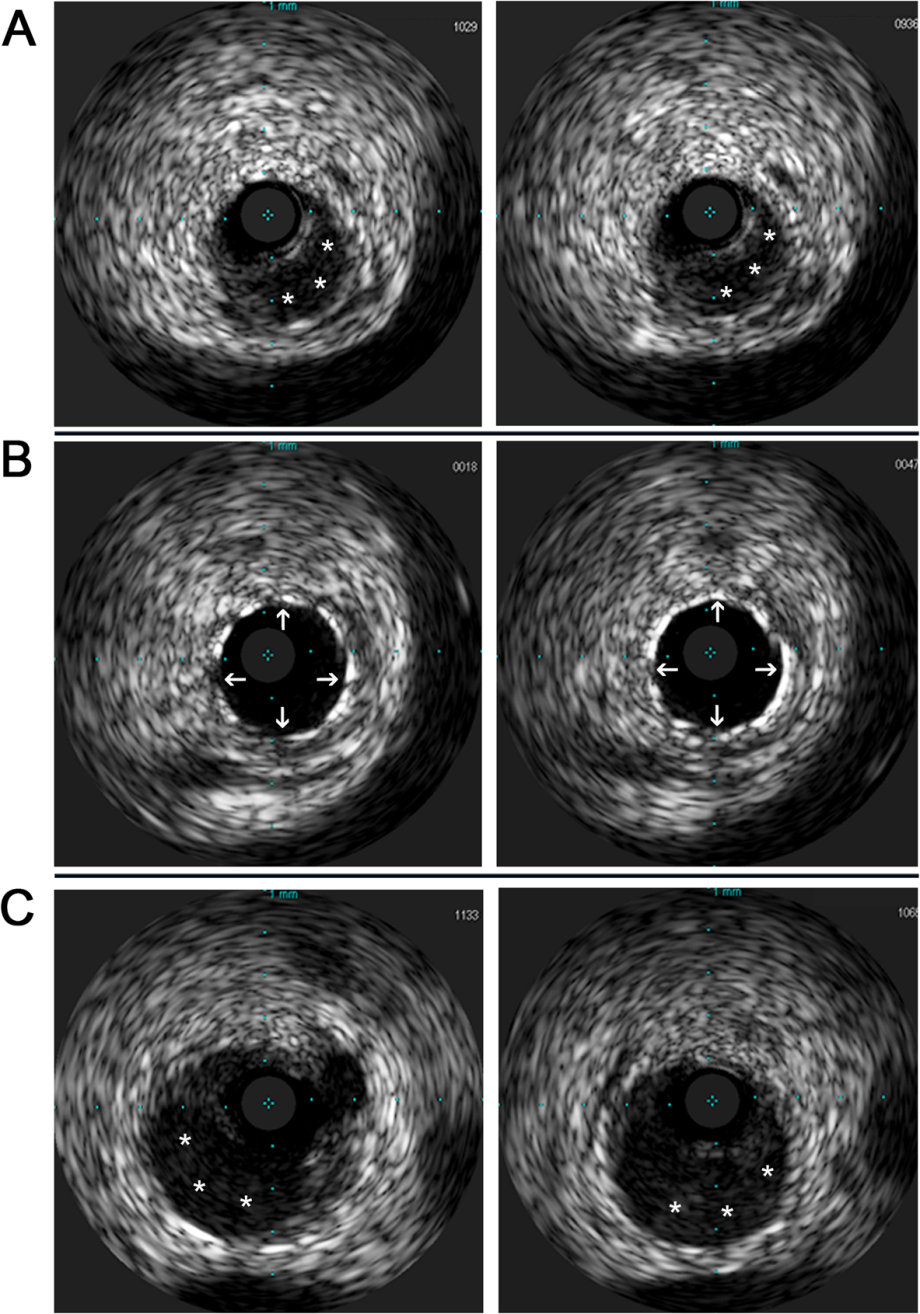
a Intravascular ultrasound (IVUS) images were presented to show the artery dissection starting from the proximal right coronary artery and ascertained the guidewire passed through the true lumen properly (left and right). b IVUS demonstrated stents attached to endothelium well and covered artery dissections completely (left and right). c IVUS showed multiple focal artery dissections located at LMC, LAD, as well as the ostium and the proximal segment of LCX (left and right). The intramural hematoma was demonstrated (*), and implanted stents were indicated with arrows
